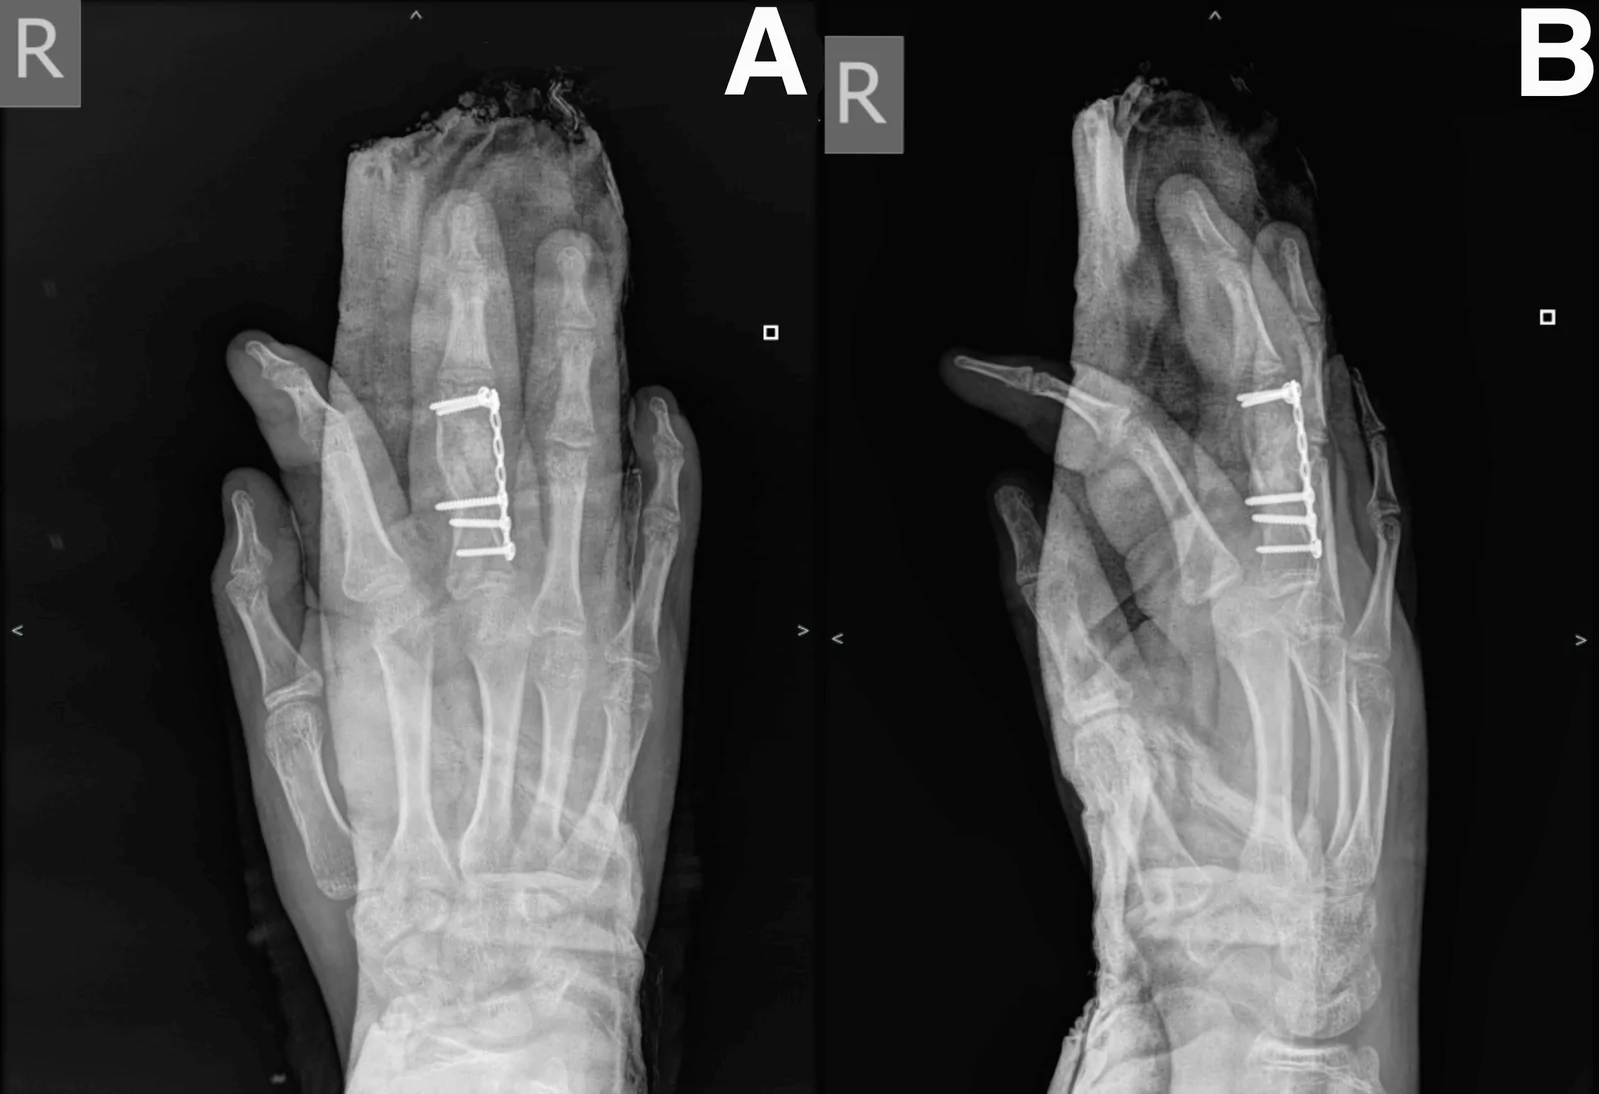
Immediate postoperative radiographs: (A) anteroposterior view; (B) oblique view.
